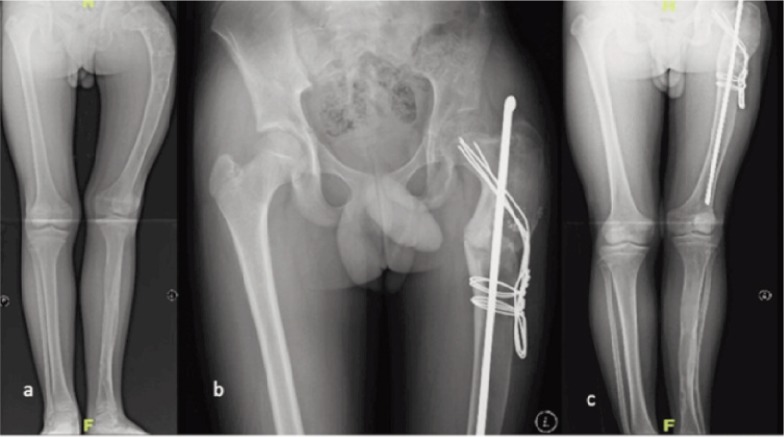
Series of radiographs of the first patient, the 14-year old boy with fibrous dysplasia: (a) Shepherd’s crook deformity of left femur with loss of the normal trabecular pattern and ground-glass appearance (b) Immediate post-operative radiograph shows valgus osteotomy and intramedullary Rush rod and Kirschner wires in the neck bent and held to side of femoral shaft distal to osteotomy site (c) The radiograph at 22 months after surgery shows the preservation of neck shaft angle with fixation devices in place.
